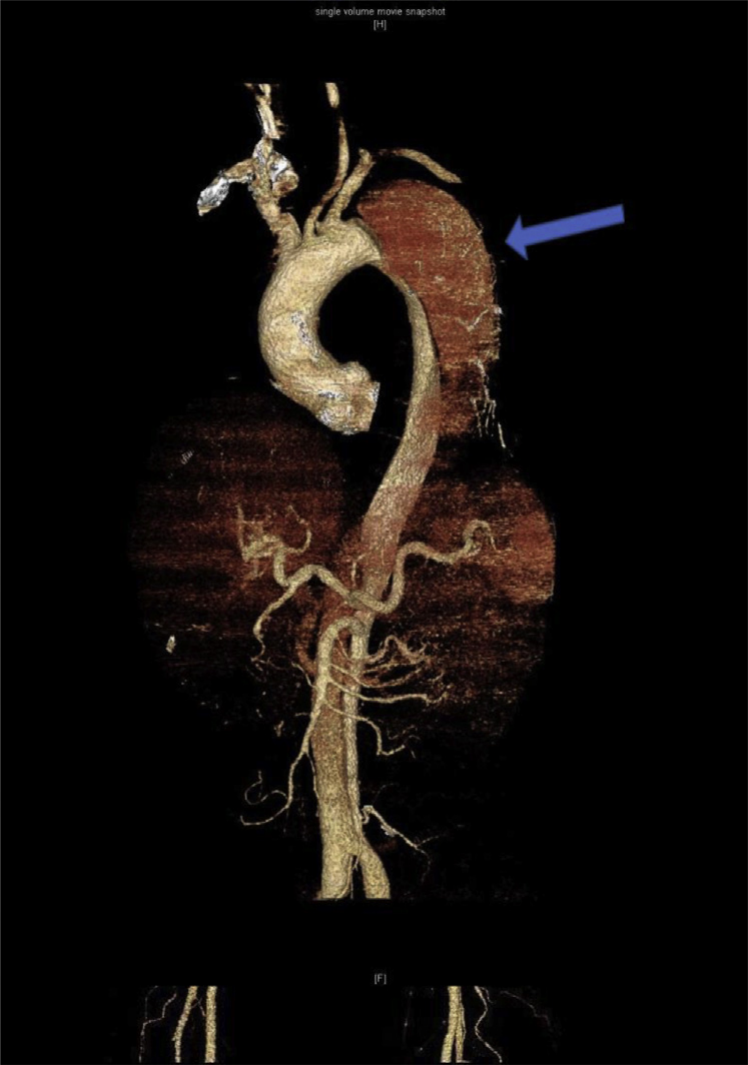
Follow-up computed tomography (CT) scan after 5 months again demonstrating type B aortic dissection (TBAD; *arrow*). The proximal descending aorta measured 6.5 cm (increased from 6.1 cm).
